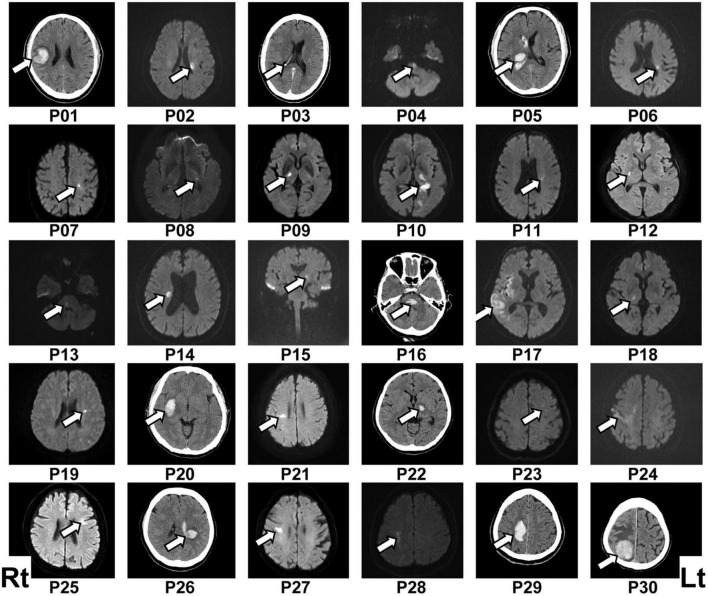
Lesion location in each patient. The patient number (Pxx) was given according to the order of participation. Lesions were located mainly by diffusion-weighted imaging following ischemic stroke (P02, P04, P06–P15, P17–P19, P21, P23–P25, P27, and P28) or computed tomography (CT) scan following hemorrhagic stroke (P01, P05, P16, P20, P22, P26, P29, and P30). The lesion location in the ischemic stroke patient P03 was determined via CT scan because of a contraindication for using diffusion-weighted imaging. The left (Lt) side of the figure represents the right (Rt) side of the brain.
